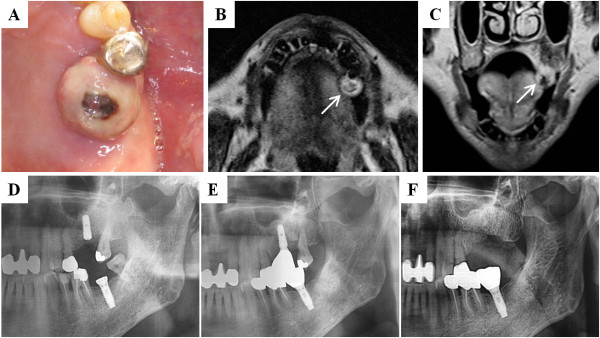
Preoperative clinical and magnetic resonance imaging views (A-C) and follow-up panoramic views for implantation (D-F). (A) A firm and round dome-shaped mass was observed in the site of the previous implant, the left maxillary first molar. (B and C) In magnetic resonance imaging, a 1.5cm heterogeneous mass was observed on the attached gingiva (arrows). (D and E) Panoramic views at 3 months (D) and 4 years (E) after fixture placement. (E) Progressive alveolar bone destruction due to peri-implantitis was observed at 4 years after implantation. (F) Panorama at the first visit to our clinic, 5 months after fixture removal. There is no associated bony defect with the gingival mass.
